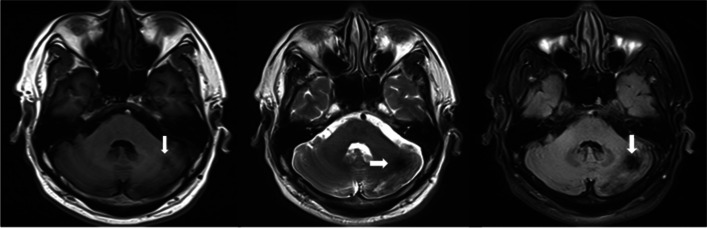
MRI scan showing the resolution of the cerebellar lesion except the traces after biopsy (arrow) after a 4-month anticoagulation therapy
